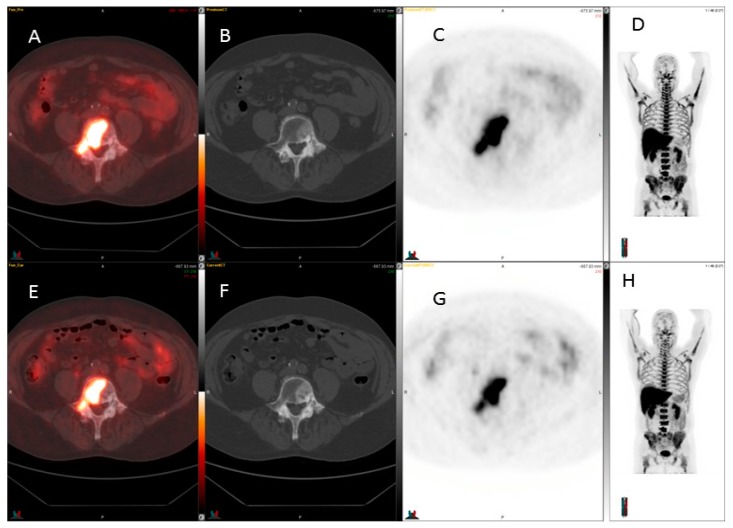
^18^F-FLT detection of prostate cancer metastases before and after therapy. The sclerotic skeletal metastases seen on CT are seen as defects on ^18^F-FLT, so the usefulness of ^18^F-FLT for detection of skeletal metastases is still unclear since visualization of lesions may be hindered by prominent background activity in the bone marrow. The skeletal metastases visualize most often as defects. Upper row, baseline: from the left fusion image (A), CT image (B), ^18^F-FLT PET image (C), and MIP image (D); lower row, after 4 week therapy: from the left fusion image (E), CT image (F), ^18^F-FLT PET image (G), and MIP image (H). Color bar range from 0 to 5.
